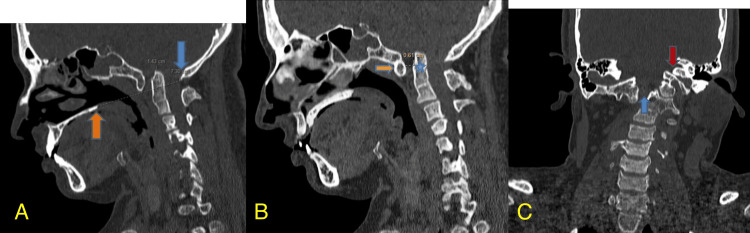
(A) Sagittal section of CT cervical spine showing basilar invagination. The tip of the odontoid process projects 14.3 mm above the Chamberlain line into the foramen magnum (orange and blue arrows indicate measurement points); (B) Sagittal section of CT cervical spine showing the atlanto-dens interval (ADI). The interval measures 6.1 mm, with the measurement points indicated by asterisks and arrows; (C) Coronal section of CT cervical spine showing non-visualization of the posterior arch of the atlas (C1 vertebra) with fusion of the posterior tubercle to the occiput (blue and red arrows indicate key structures).
